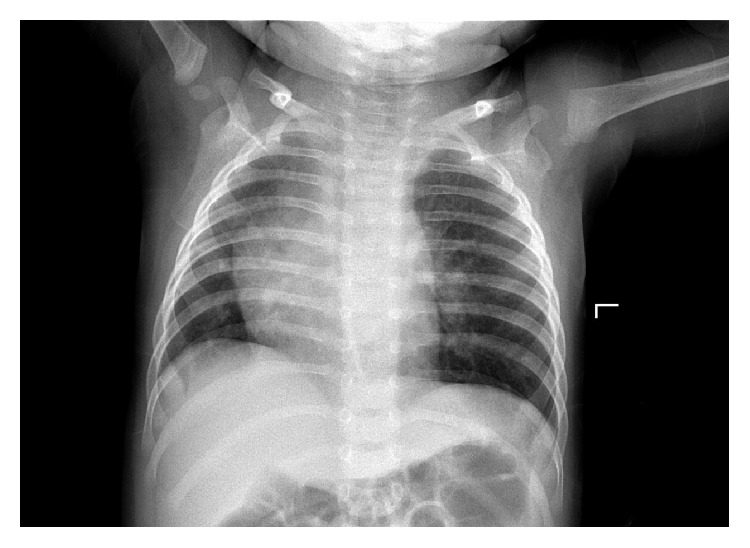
Chest X-ray showing continued presence of bronchopneumonia, mediastinum right shift, and emphysema on the left-hand side.
